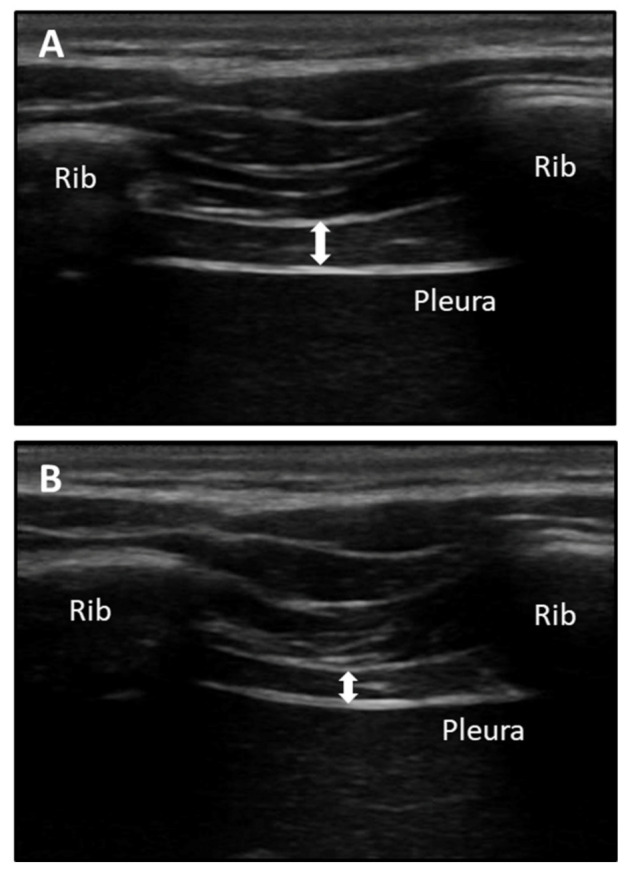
B-mode diaphragm thickness ultrasound imaging visualizing the last intercostal space following the mid-axillary line from the inferior edge of the 11th rib to the superior edge of the de la 12th rib of the thorax. (A) Diaphragm thickness (white arrow) at maximum inspiration (T^ins^) during relaxed breathing. (B) Diaphragm thickness (white arrow) at maximum expiration (T^exp^) during relaxed breathing.
